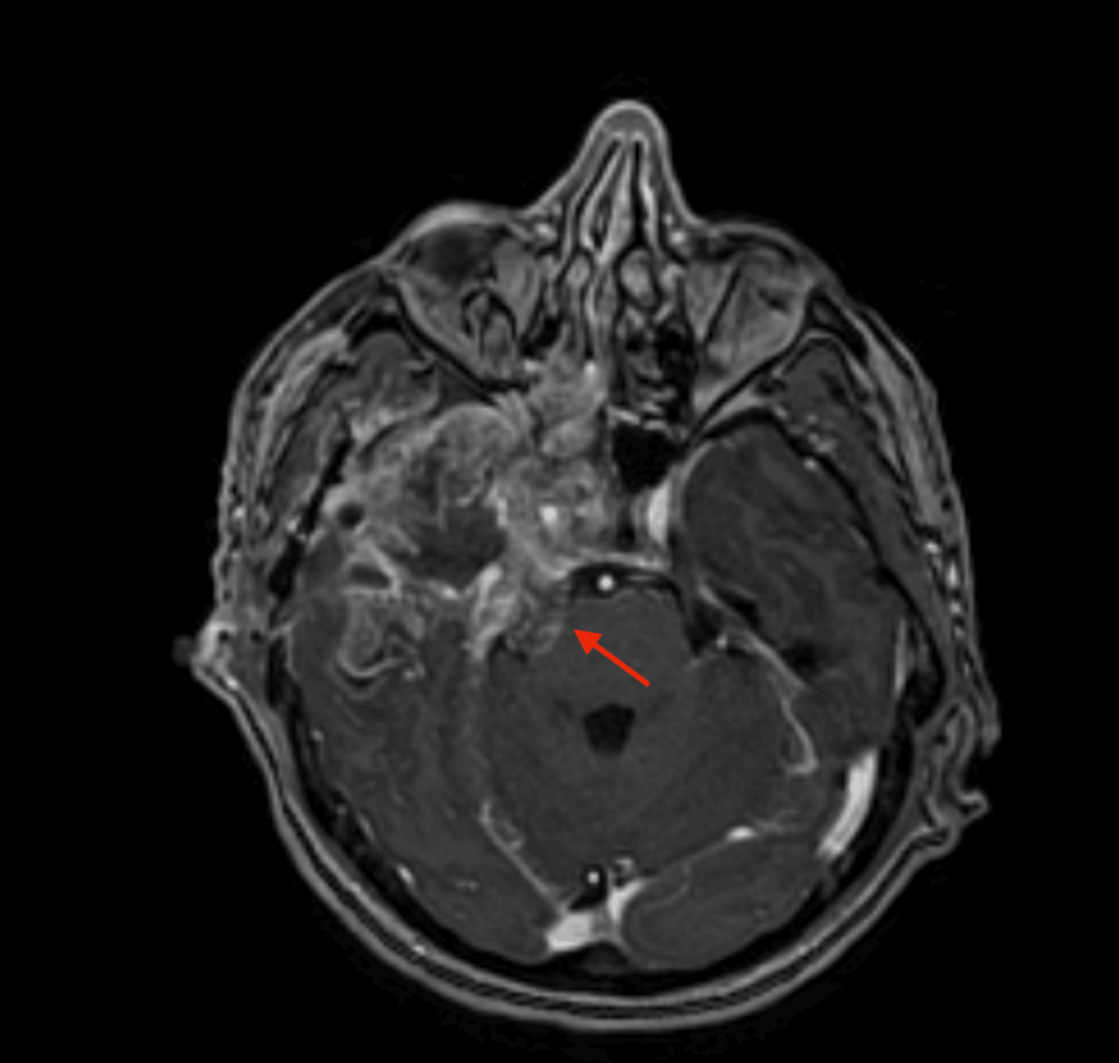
MRI of the brain The red line points at the tumor spread to the right cerebello-pontine angle.
